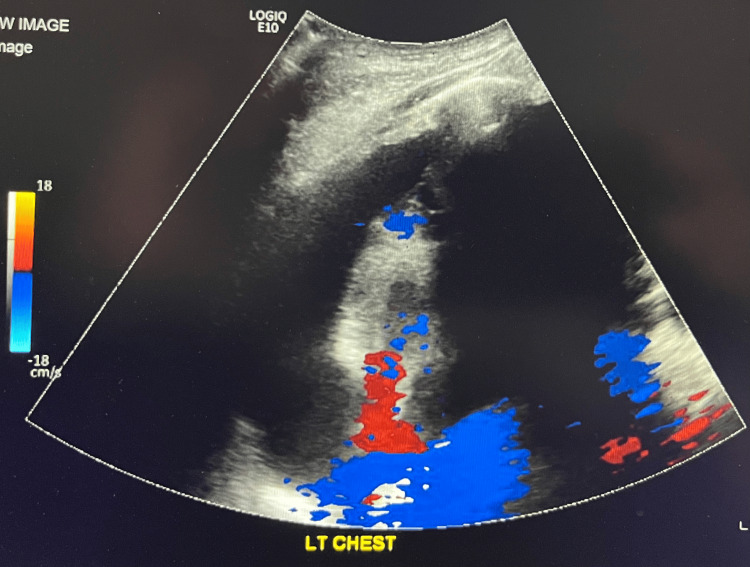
Preoperative ultrasound of the pleural space with color Doppler
